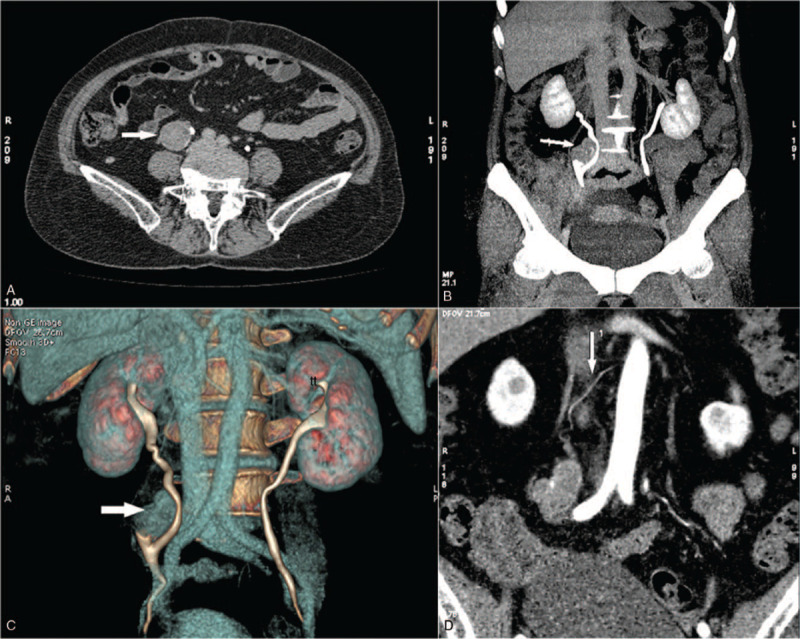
Computed tomography (CT) demonstrates a dysplastic supernumerary kidney (arrow) under the normal right kidney (A–C), and a separate arterial supply (arrow) originating from the aorta (D).
